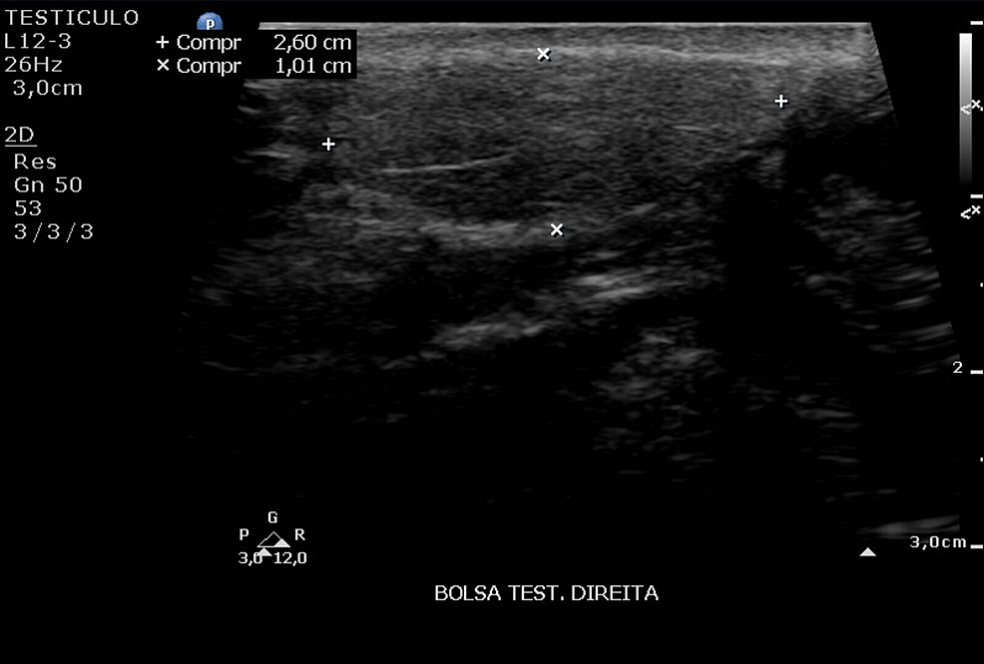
Ultrasound of the right testicular pouch Presence of nodular image, measuring 2.6 x 2.1 x 1.0 cm, isoechogenic to adipose tissue
